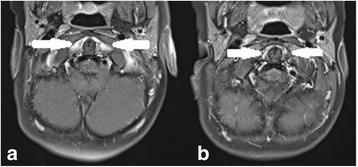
MRI of cervical spine (patient #5) (T1 TSE FS axial with a contrast medium): a inital MRI showed an intense contrast enhancement of the thickened synovia in pre- and paradental space (white arrows), b follow-up MRI 6 months later showed a reduction of synovial thickening and a significantly less intense enhancement (white arrows)
